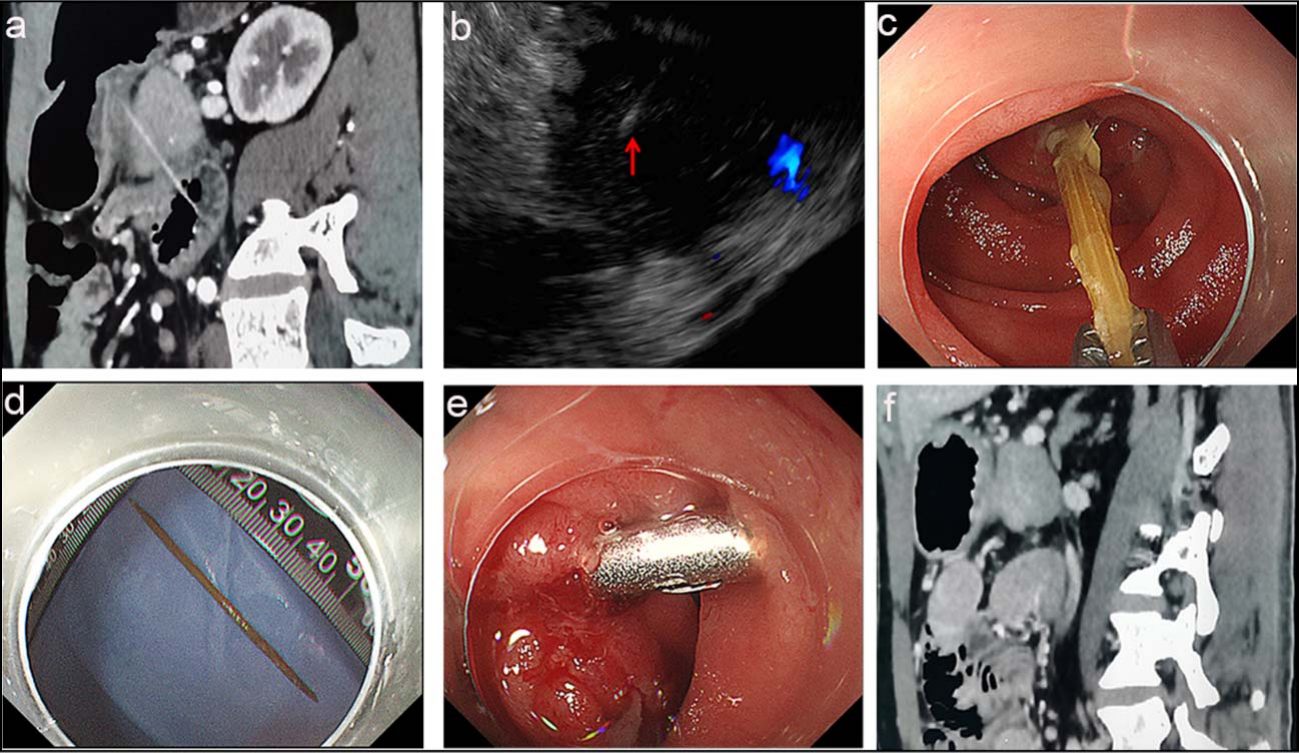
CE-CT indicated that a 6-cm foreign body traversing from the pancreas head to the small intestine (a). The red arrow indicates a long, thin, hyperechoic foreign body crossing the pancreas as confirmed by endoscopic ultrasonography (EUS) (b).The toothpick was removed under enteroscopy using foreign body forceps (c). The toothpick image (d). The postoperative wound was completely closed by endoscopic clips (e). CE-CT 3 days later showed no significant complications (f).
